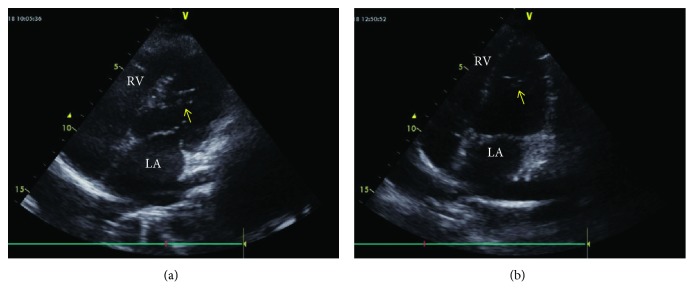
B-mode echocardiographic image from the father (a) and his daughter (b). (a) and (b) Modified apical four chamber view by B-mode recording. The arrow indicates the false tendon stretching from the left ventricular apical free wall to the ventricular septum. LA: left atrium; RV: right ventricle.
